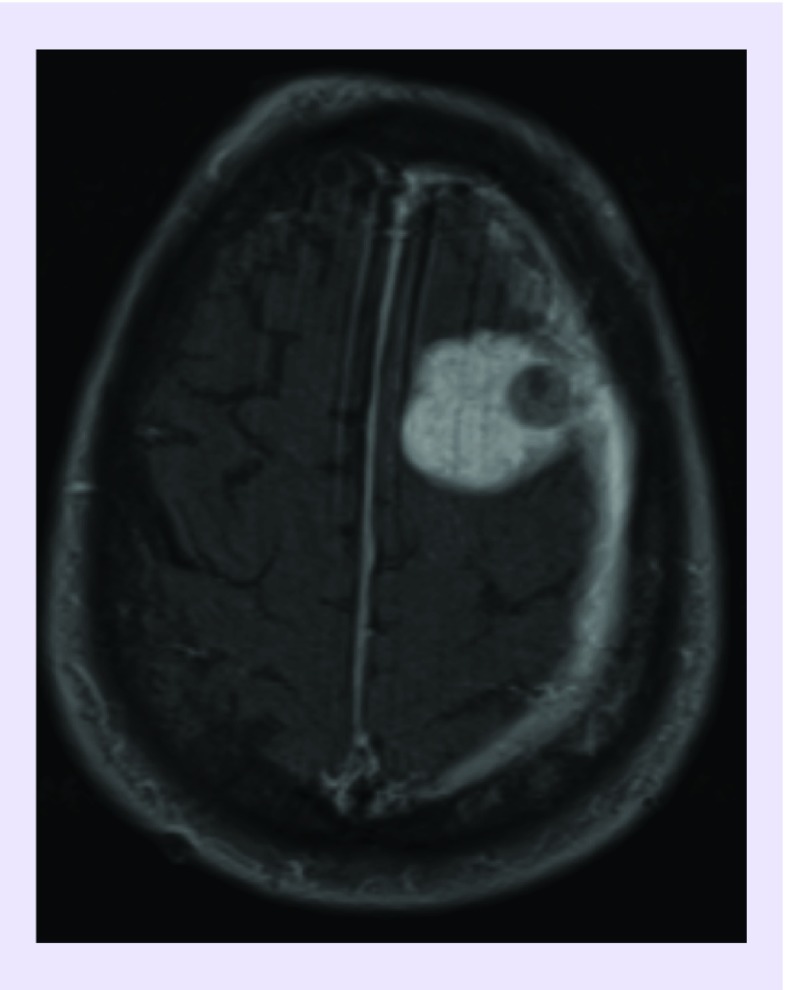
Axial T1 contrast-enhanced MRI. An axial T1 contrast-enhanced MRI image demonstrates a large left frontal enhancing mass. Within the lesion is a small, round nonenhancing mass.
